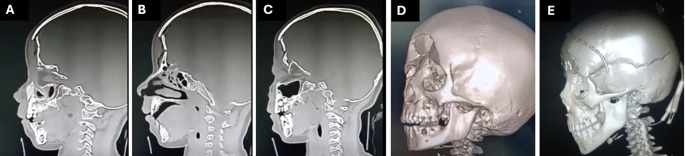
CT scan of depressed frontal sinus/bone fracture; A, B, C. sagittal cuts showing fractured orbital roof in A and C with entrapped bony spicules inside the orbit in C, fracture ethmoidal roof in B; D, E preoperative versus postoperative 3D CT scan showing improved projection after surgery
